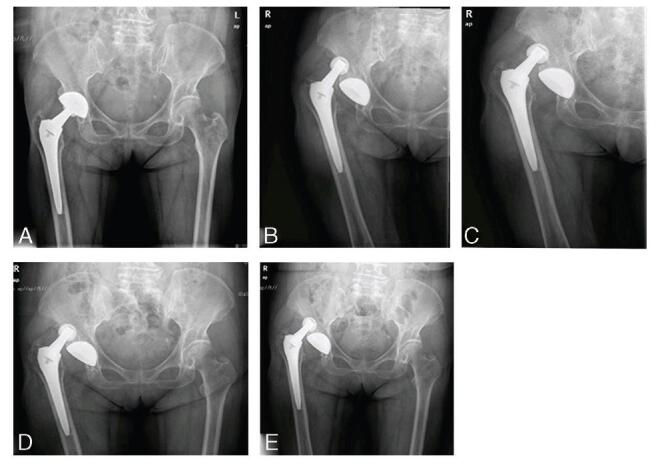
Figure 1. X-ray radiography of the artificial hip before the first revision. A, X-ray of the hip after primary hip arthroplasty. B, X-ray of the first dislocation of artificial hip. C, X-ray of the second dislocation of artificial hip. D, X-ray of the third dislocation of artificial hip. E, X-ray of the fourth dislocation of artificial hip.
